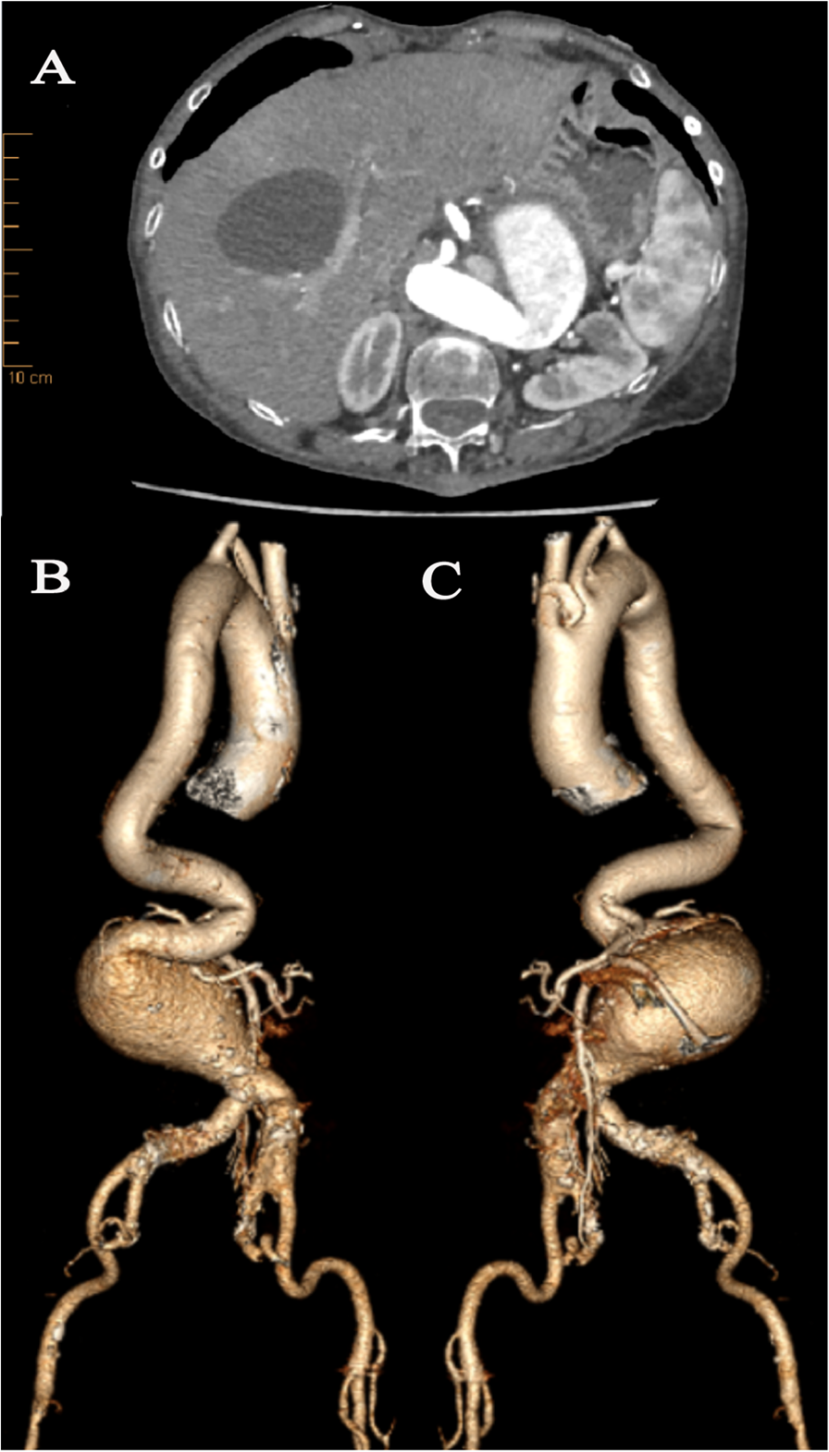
Preoperative CTA and 3-dimensional reconstructions. (A) Preoperative CTA showed the infrarenal AAA with tapered neck and severe angulation in axial view. (B, C) 3-dimensional reconstructions confirmed severely tortuous descending aorta and infrarenal AAA with really hostile neck. Aneurismal dilatation and severe calcification of bilateral iliac arteries and tortuosity of left aortoiliac access were observed.
